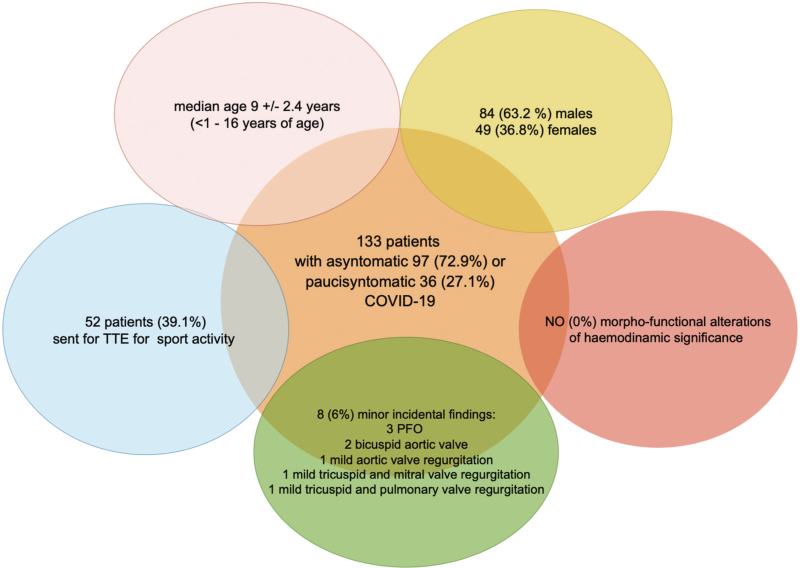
Indications for transthoracic echocardiography, and data of patients and findings of transthoracic echocardiography.
